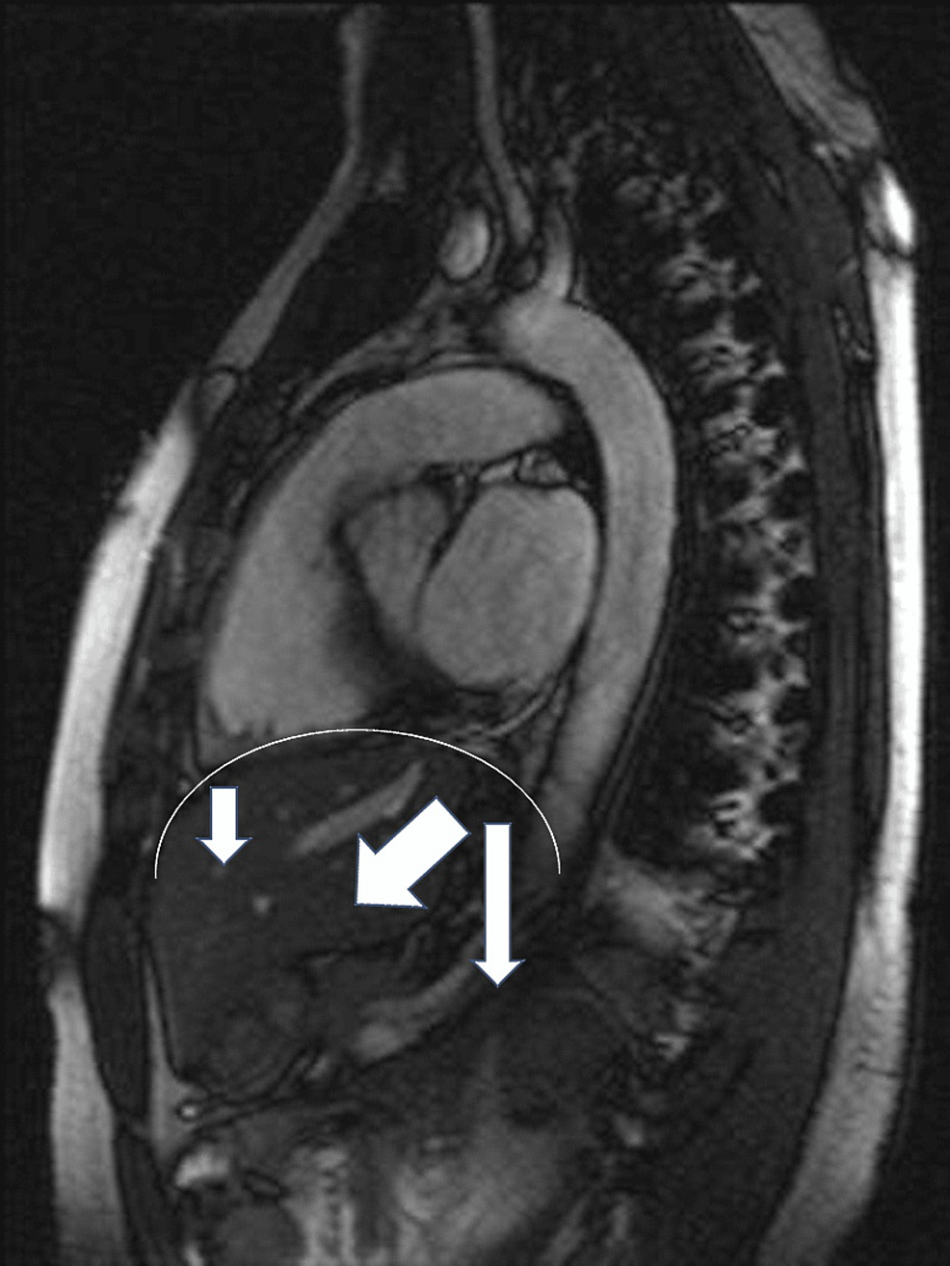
The image shows the position of the diaphragm in a healthy subject (curved white line). The examination is done with magnetic resonance imaging in the sagittal section. The white arrows indicate the direction of the diaphragm during any inhalation (oblique, anterior, and caudal). The image is the property of Bordoni Bruno (there is the patient's consent for the use of this figure).
